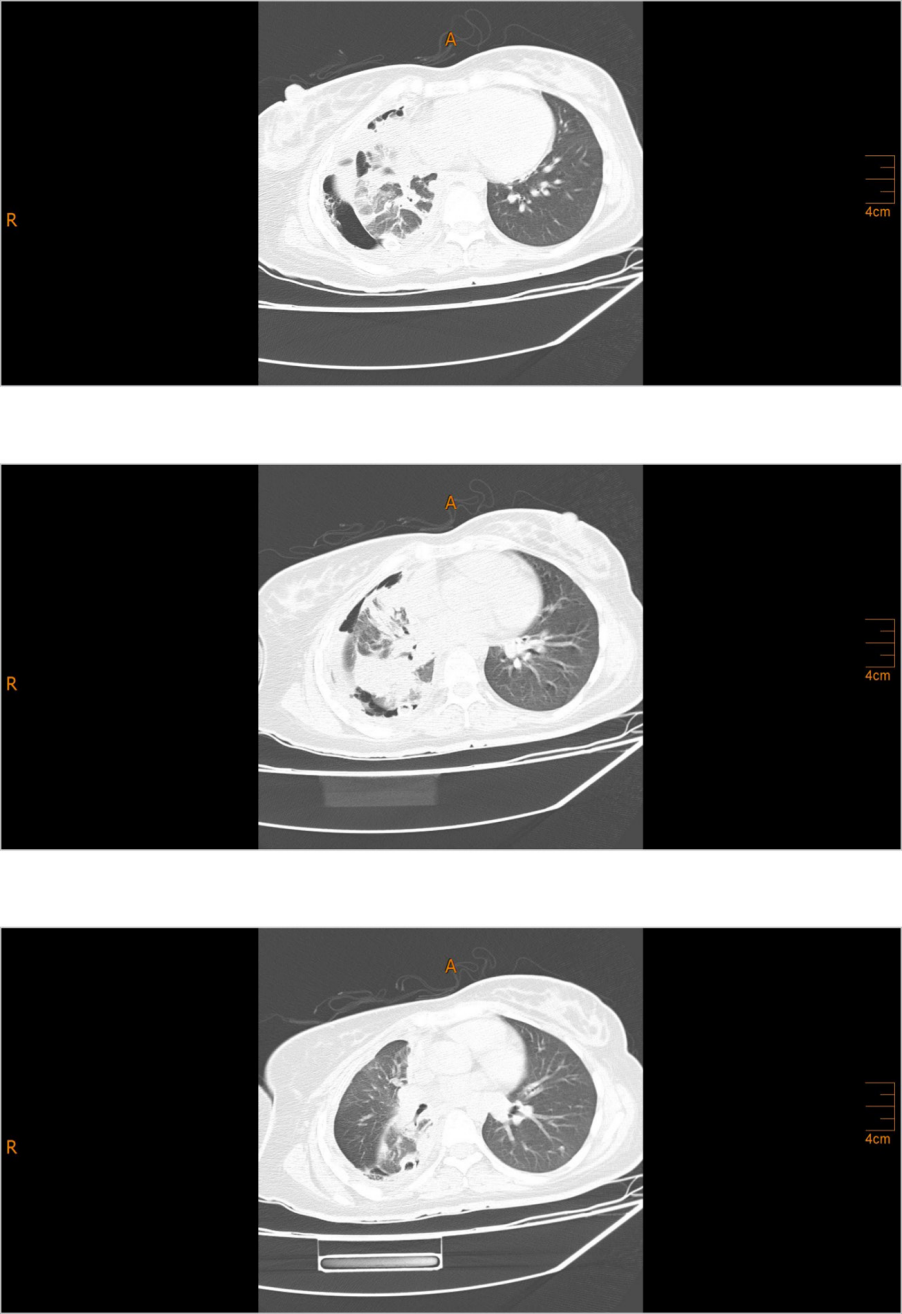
2022/04/17—spiral lung CT showing pleural effusion plus pyopneumothorax and consolidation causing the collapse of the RLL in the right hemithorax.
